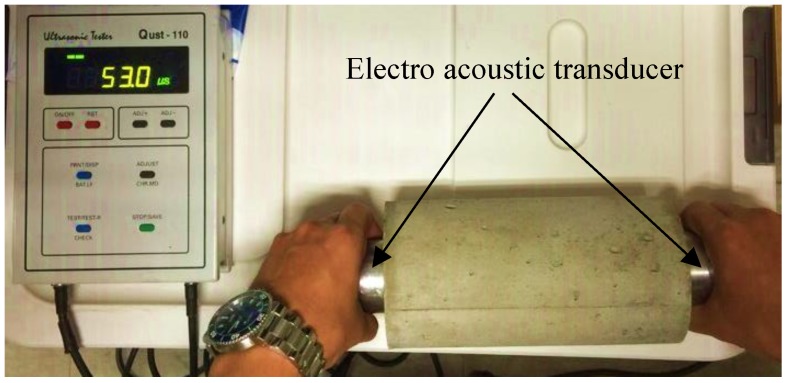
Ultrasonic pulse velocity test.
